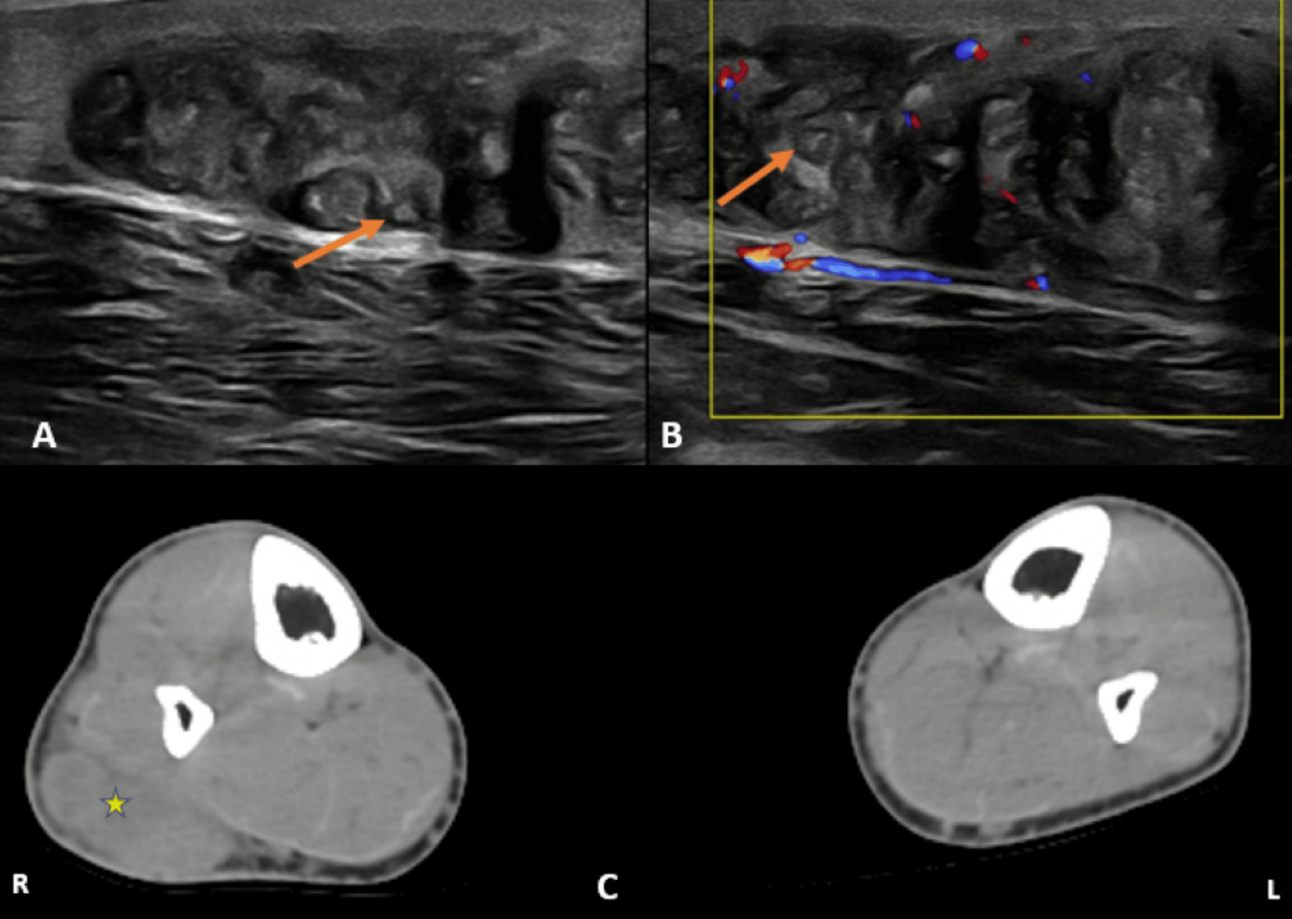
On ultrasound, a well-defined, heterogeneous, predominantly hypoechoic lesion of size 1.8 x 5.1 x 7.1 cm was noted in the subcutaneous plane in the mid-calf region, at the postero-lateral aspect of the right leg. (A) A few round hypoechoic areas with hyperechoic central foci were observed (orange arrow). (B) On color Doppler, it showed focal areas of vascularity. (C) On further investigation, non-contrast axial CT showed a well-defined, lobulated, heterogeneous, predominantly isoechoic soft tissue mass lesion at the postero-lateral aspect of the right leg, in the subcutaneous plane with mild adjacent fat stranding (yellow star). No evidence of any surrounding fluid attenuation or discharging sinuses was noted.
